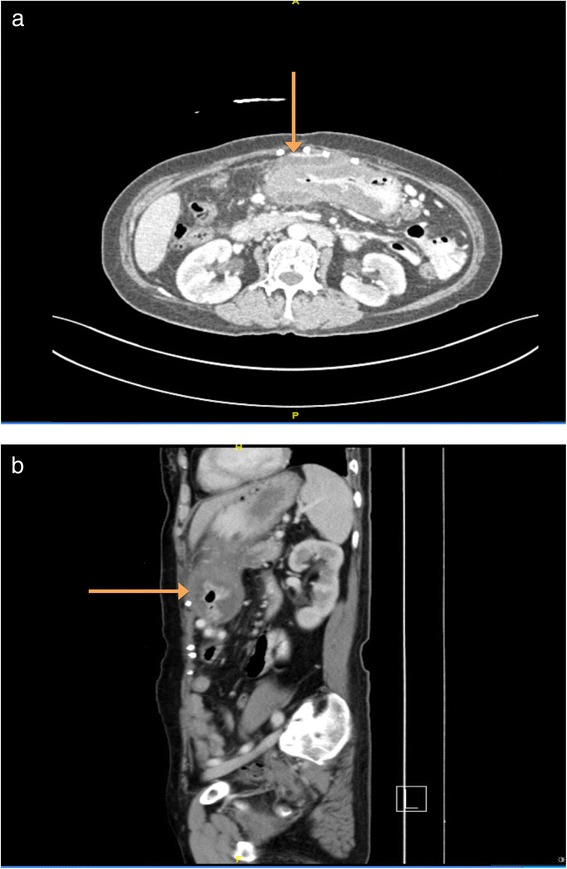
(a and b) CT imaging (transverse and sagittal views) showing thickening of the body and pylorus of the stomach (orange arrow) and a thin soft tissue plane separating the stomach lesion and the pancreas. The appearance of tissue extending between the two abdominal wall tacks suggested a recurrence of the incisional hernia but this was found not to be the case intraoperatively. Intraoperatively, the mesh was found to be adherent to a loop of small bowel
